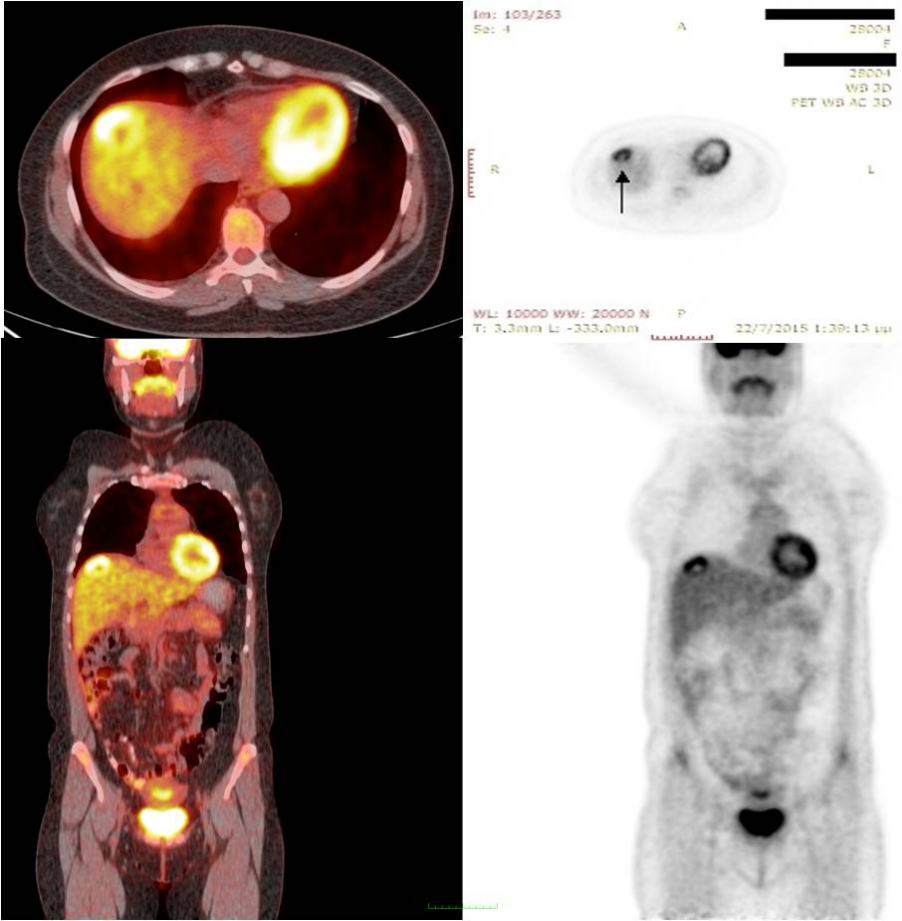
Eighteen PET/CT scans reveal that the lesion is hypermetabolic with a standardized uptake value (SUVmax) of 8.
